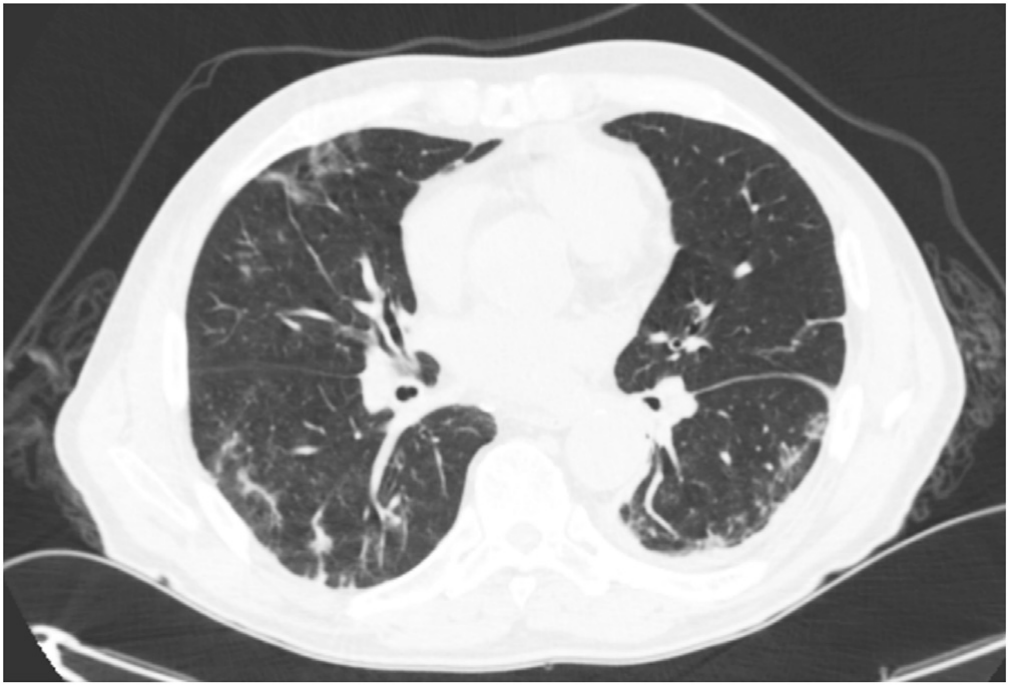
Axial CTA image showing small patchy ground-glass opacities in bilateral pulmonary bases.
